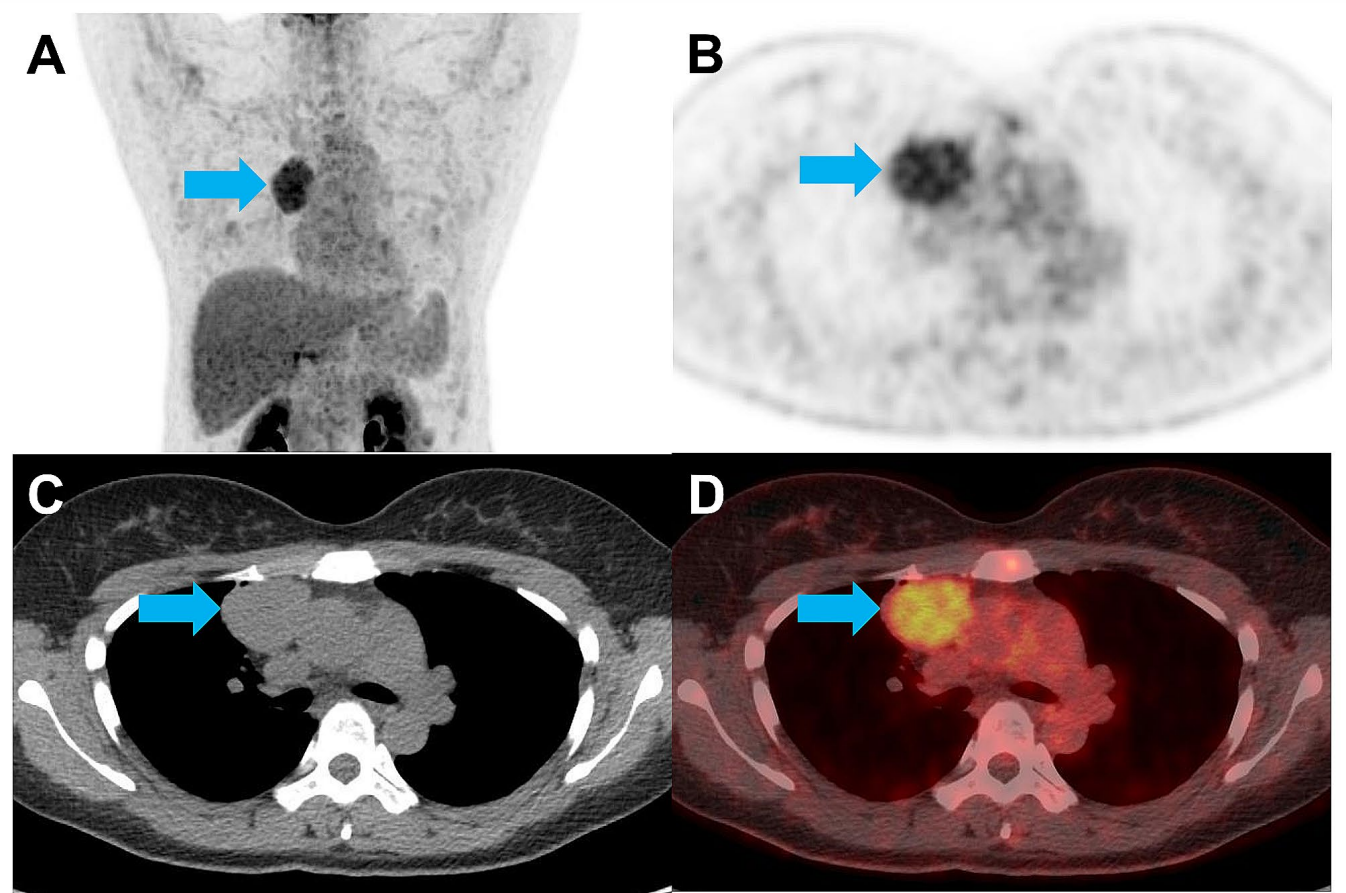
Representative [^18^F]FDG PET/CT from a 38-year-old woman with low-risk thymoma (blue arrows). (A) Maximal intensity projection and (B) axial PET images show an anterior mediastinal mass with grade 4 uptake, no central metabolic defect, and SUVmax of 3.9. (C) Axial CT demonstrates an anterior mediastinal mass with a round shape, diameter of 4.4 cm, and attenuation of 42.7 HU. (D) Axial PET/CT fusion image
